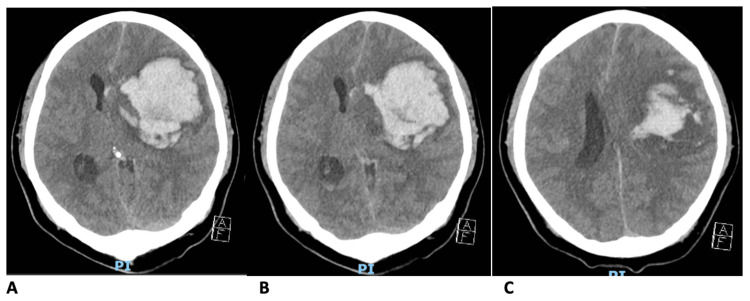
Patient’s CT brain on admission. A-C: Urgent CT brain showed large left basal ganglia hematoma with intraventricular extension, mass effect causing effacement of the left lateral ventricle and midline shift.
